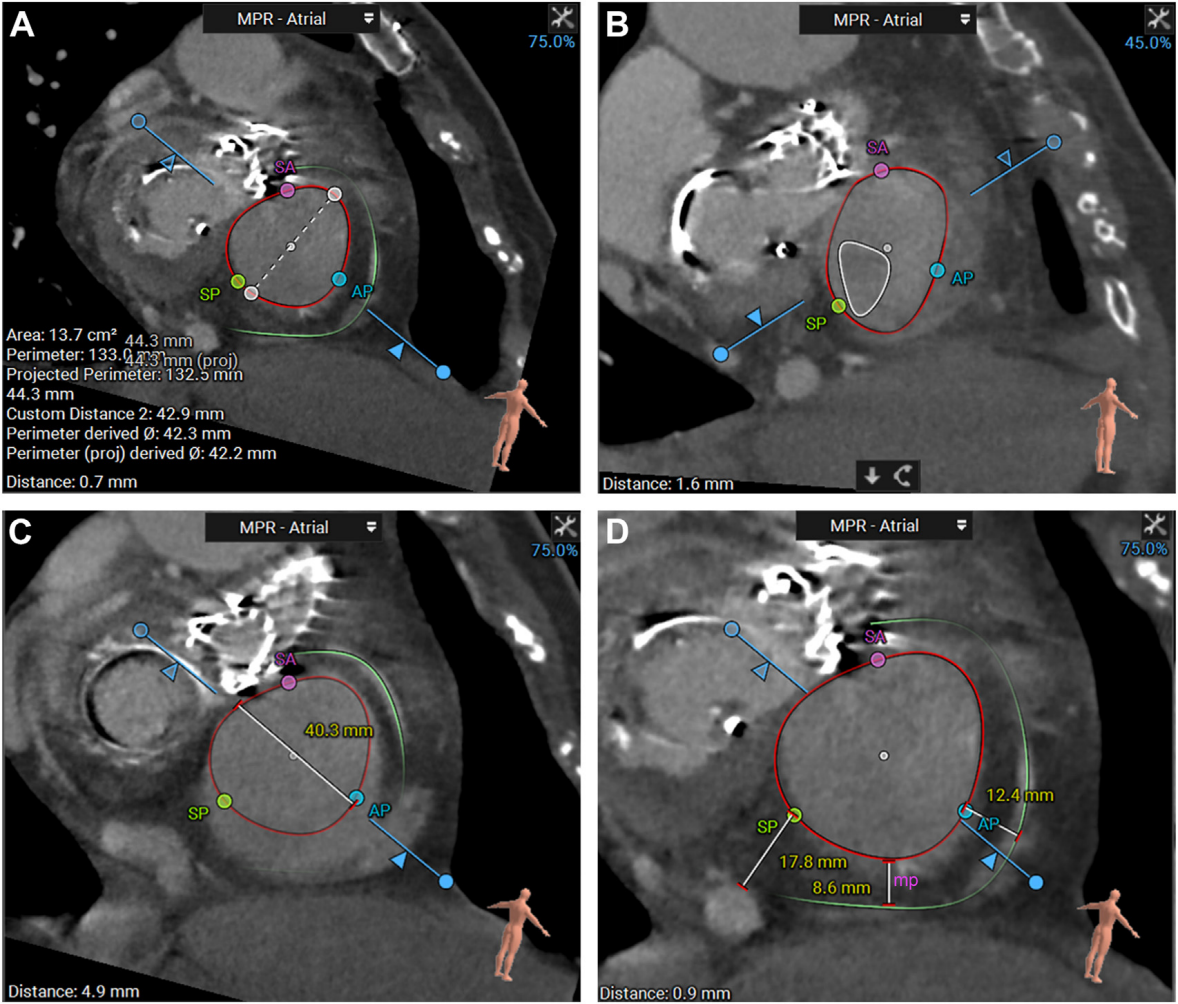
Preprocedural CTA (A) Tricuspid valve orifice area and tricuspid annulus circumference in diastole. (B) TR orifice shape in systole. (C) Septolateral diameter of the tricuspid valve annulus in diastole. (D) Annulus to right coronary artery distance in the location of the posteroseptal commissure (sp), the midpoint of the posterior valve annulus (mp), and anteroposterior commissure (ap), respectively. CTA = computed tomographic angiography; TR = tricuspid regurgitation.
